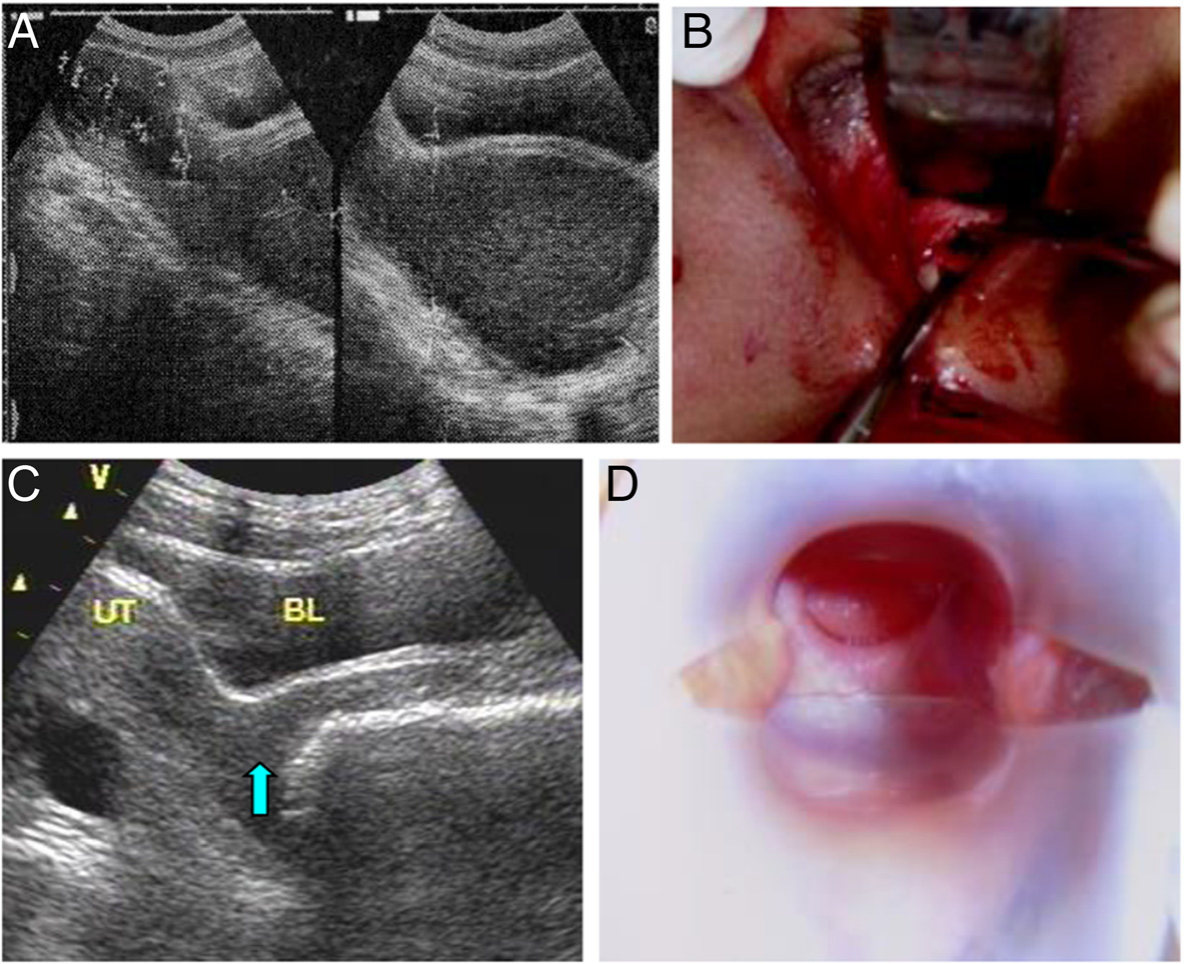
Type I cervical atresia before, during, and after tracheloplasty. (A) Ultrasonic image of Type I congenital cervical atresia. (B) After the hematoma was discharged, the uterine isthmus cysts became smaller, and the wall consisted of 5 to 6 mm of muscle tissue. (C) Fifteen days after the operation, the volume of the uterine isthmus measured 2.1 cm?×?1.5 cm. The arrow in the figure points to the vagina outside the cervix. (D) Long-term follow-up showed that the cervix resembled that of a normal nulliparous woman.
